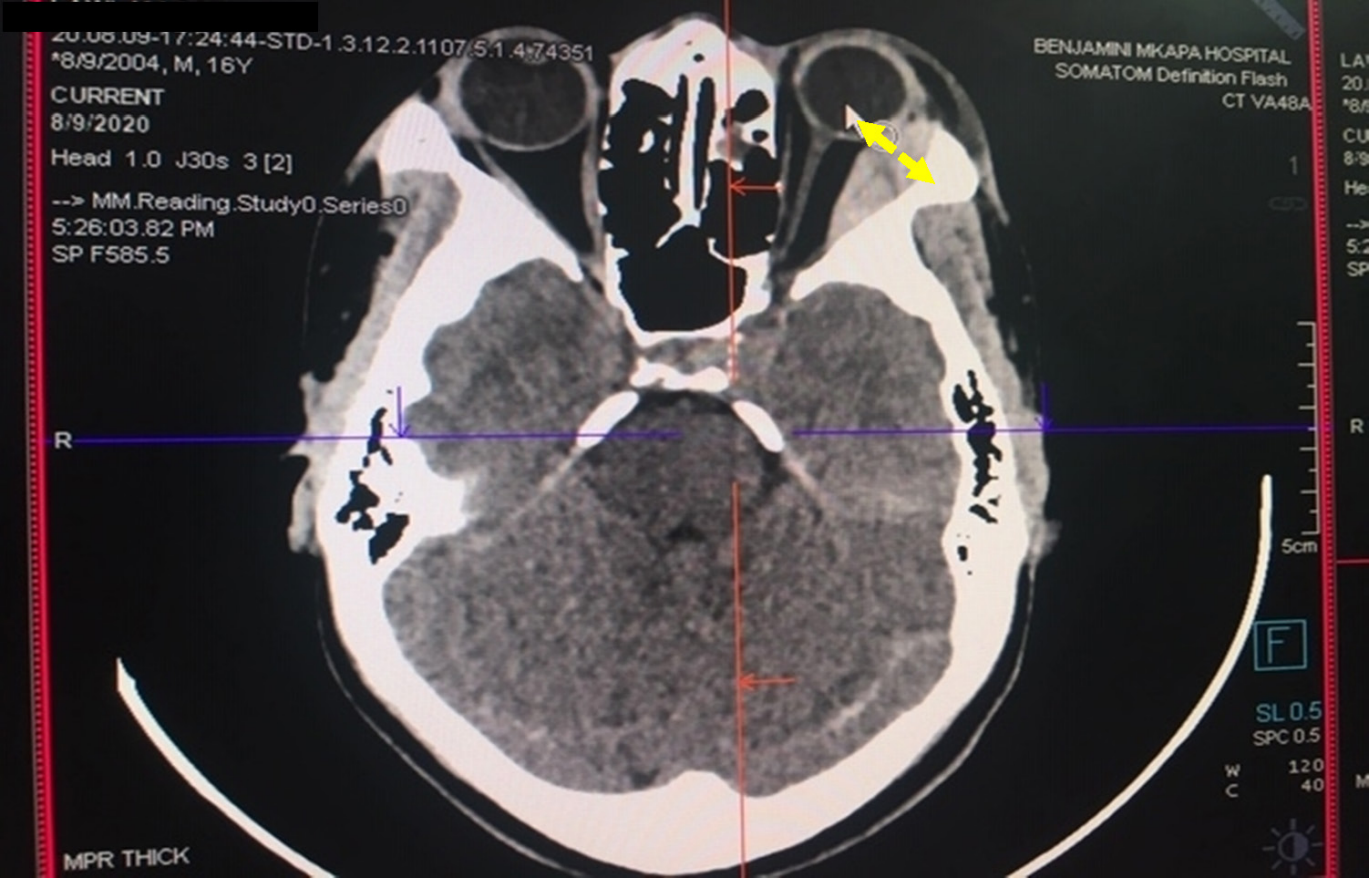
Enhanced head CT scan axial view showing left orbital hematoma with orbital pressure exertion (yellow arrow) and intact calvarium and normal brain parenchyma.
